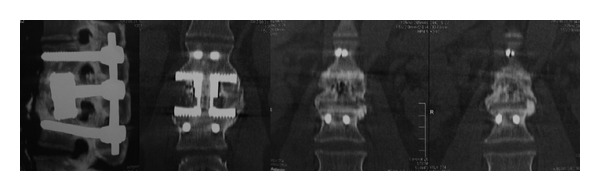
CT scan showing solid fusion 24 months after the procedure. The device design promotes the ossification inside and outside of the prosthesis.
